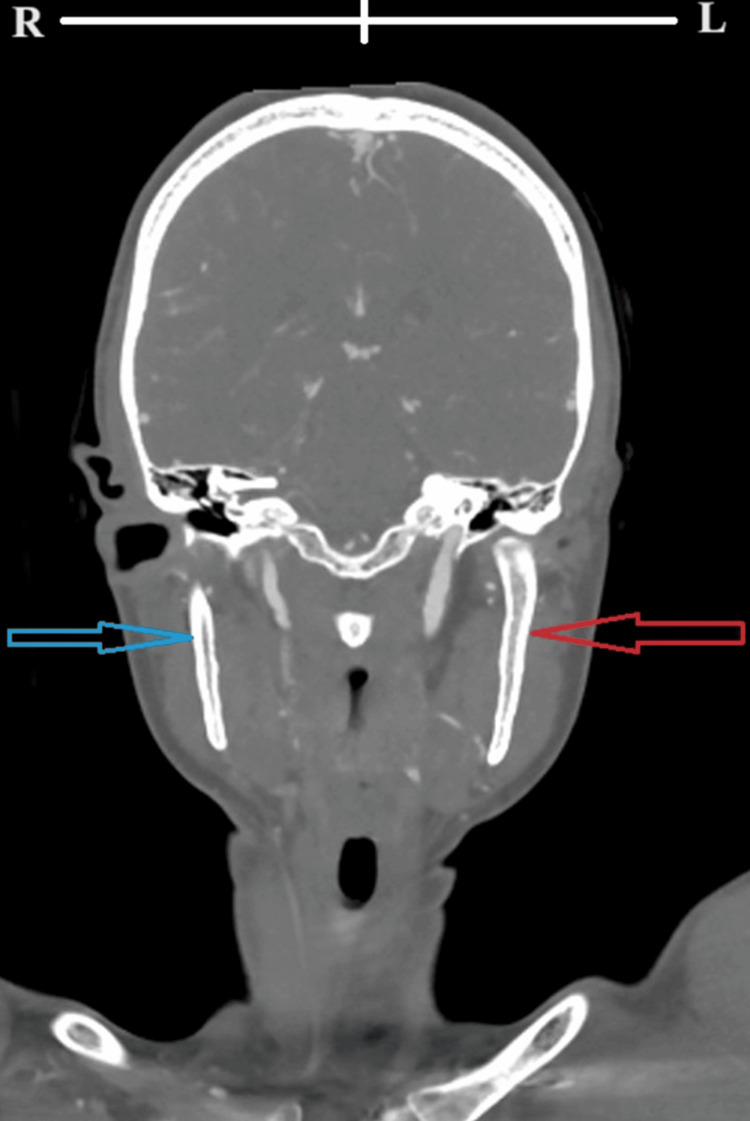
A follow-up coronal computed tomography angiogram of the neck showing normal left internal carotid artery (red arrow) and mild residual right internal carotid stenosis (blue arrow). R: right; L: left
